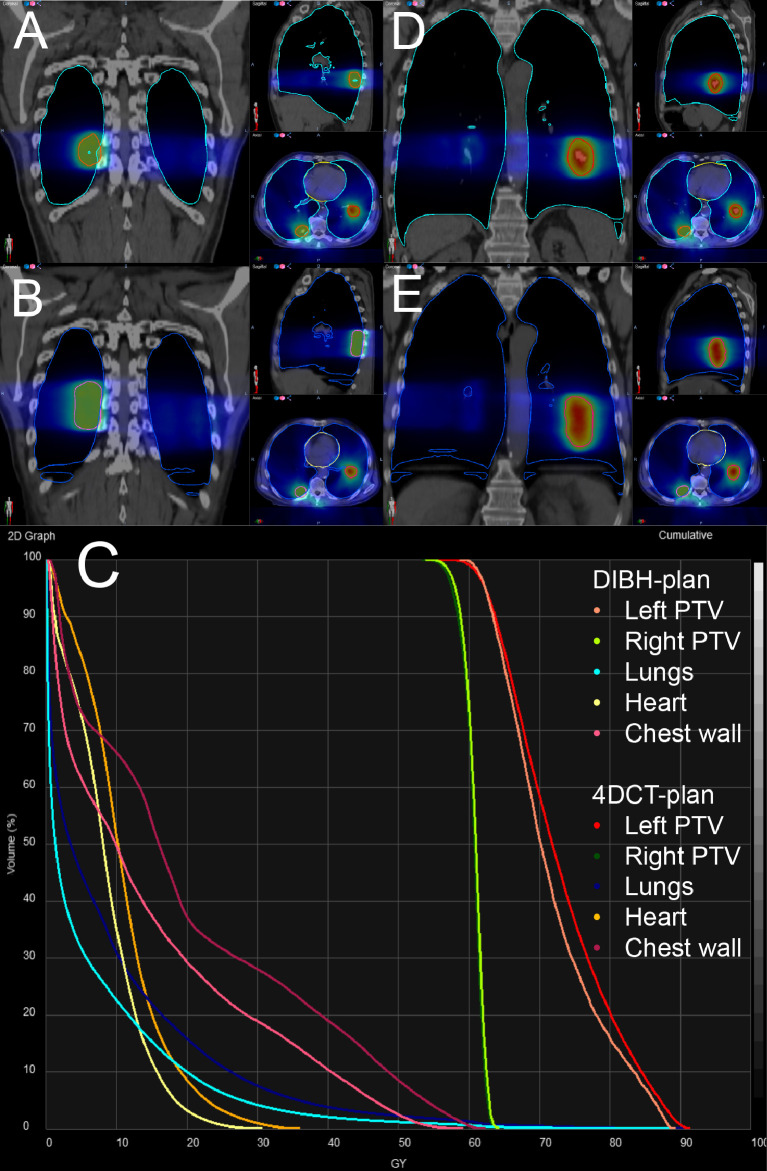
Dose distribution of the summed deep inspiration breath hold (DIBH) plans (A, D) versus 4-dimensional computed tomography (4D-CT) plans (B, E) and dose-volume histogram (C) of DIBH and 4D-CT structures. The curves of the right planning target volumes (PTVs) in DIBH and the 4D-CT plan are almost congruent.
